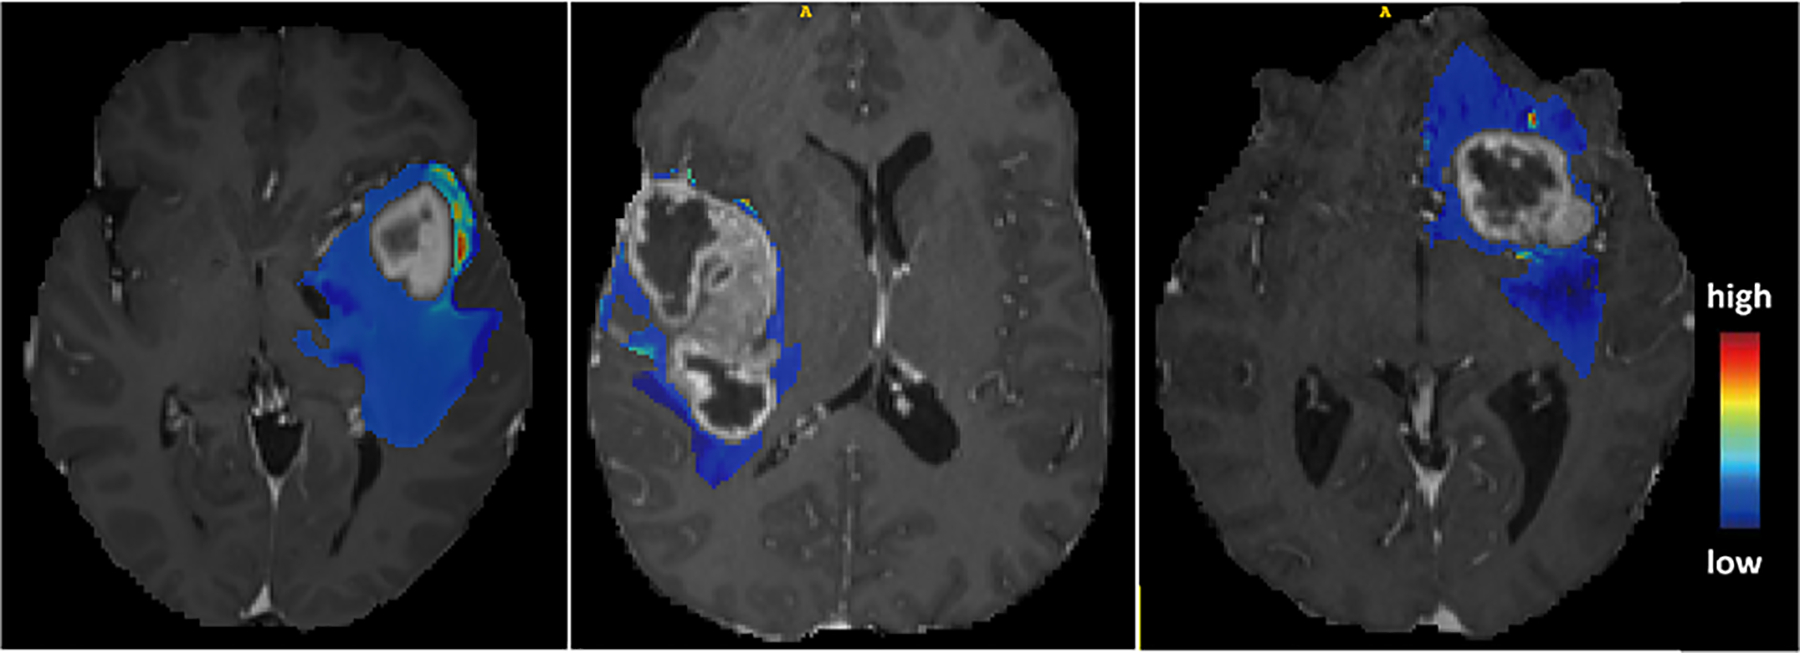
The *predictive maps* overlaid onto patients’ MRI scans. The *predictive maps* are color-coded to indicate high or low probability of tumor infiltration.
